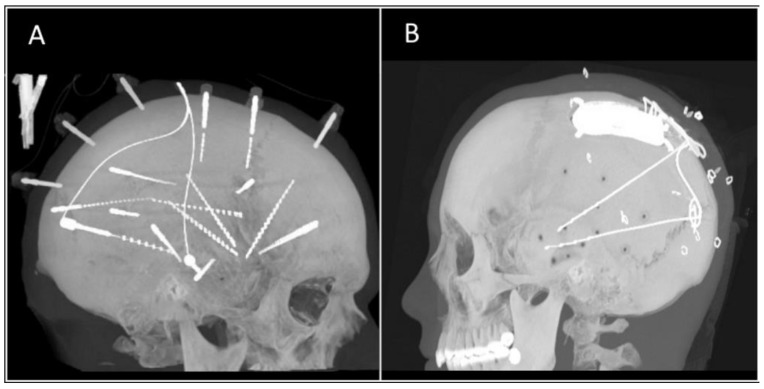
Computed tomography (CT) reconstruction images of patients after implantation of (A) sEEG electrodes and (B) RNS system.
